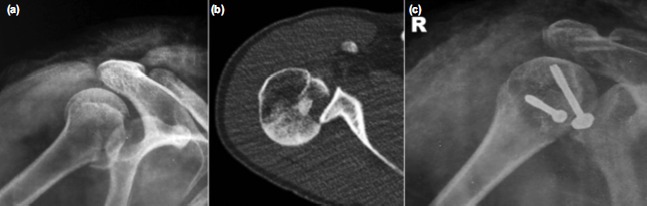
(a) Pre-op radiograph, (b) Pre-op scan axial section and (c) Post-op radiograph of right shoulder.
